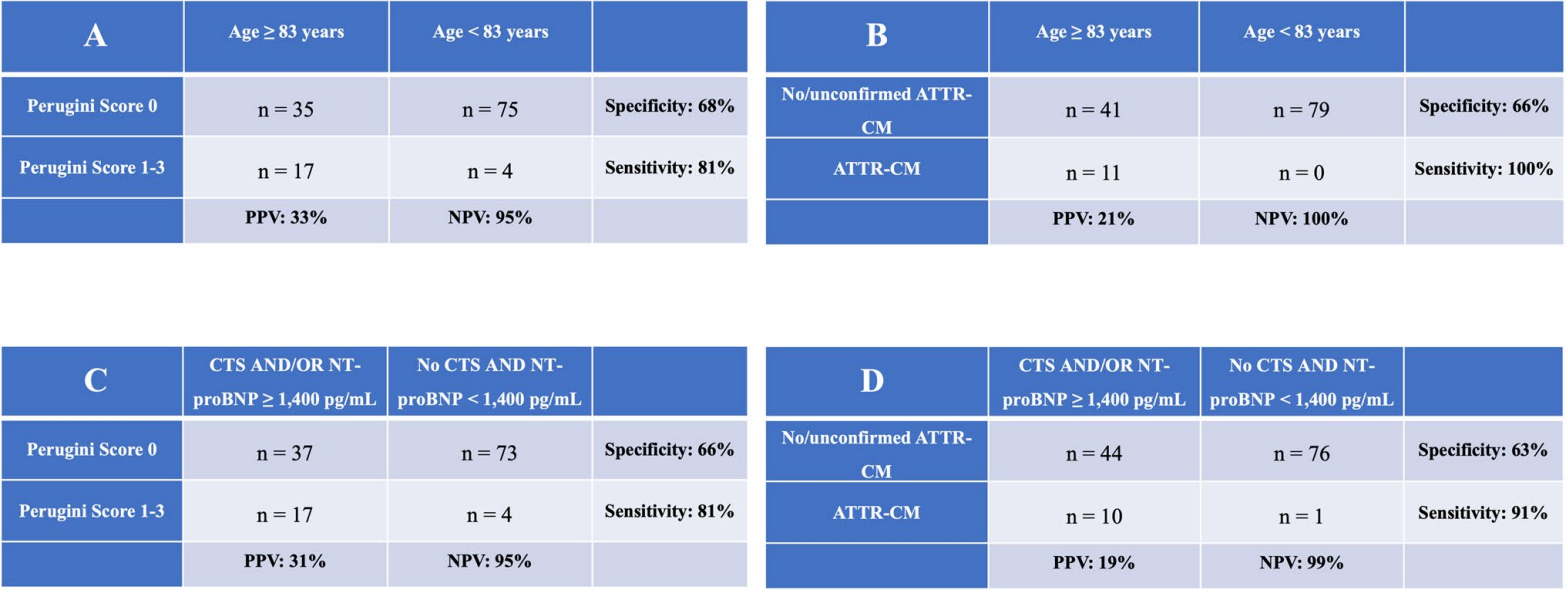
Sensitivity, specificity, PPV, and NPV for age ≥ 83 years (A and B) and the combination of CTS and/or NT-proBNP ≥ 1,400 pg/mL (C and D) regarding pathological bone scintigraphy result and definitive ATTR-CM diagnosis
